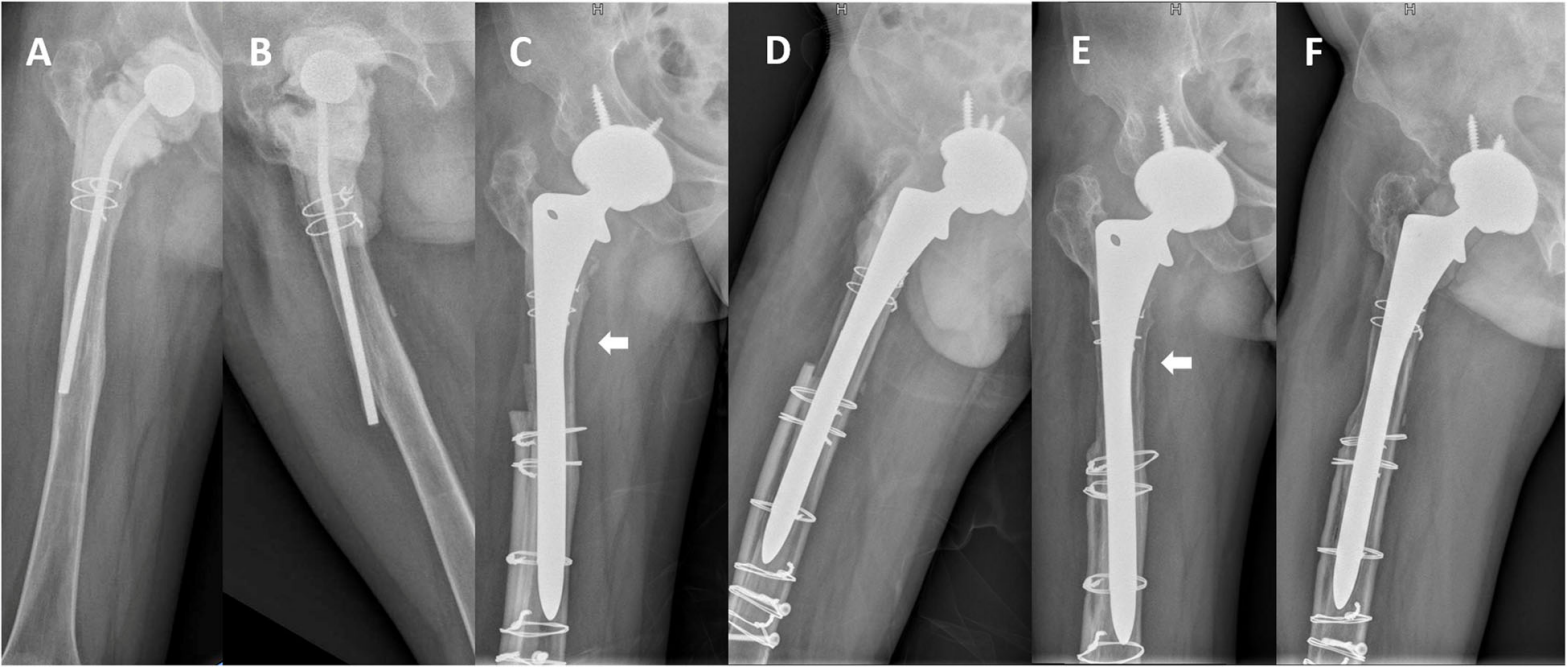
a-b Preoperative radiographs of a 38-year-old man who underwent revision THA for periprosthetic joint infection, showing the placement of antibiotic-loaded spacer with Paprosky type IIIA femoral bone defect. c-d Radiograph immediately after revision with extensively porous-coated stem and cortical strut allografts. e-f Radiographs at 9 years after revision. Bone ingrowth and no signs of stem loosening were observed. Cortical strut allografts incorporated to the host bone successfully. Moderate stress shielding was observed in proximal femur both medial and lateral sides (white arrow)
